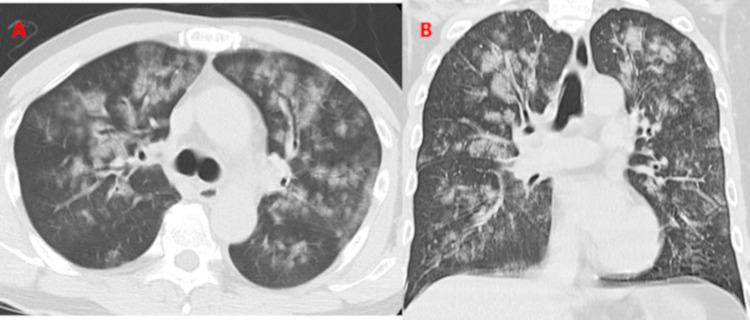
Chest computed tomography on admission Computed tomography revealed diffuse ground-glass opacity in the bilateral lung large (A) axial view (B) coronal view.
